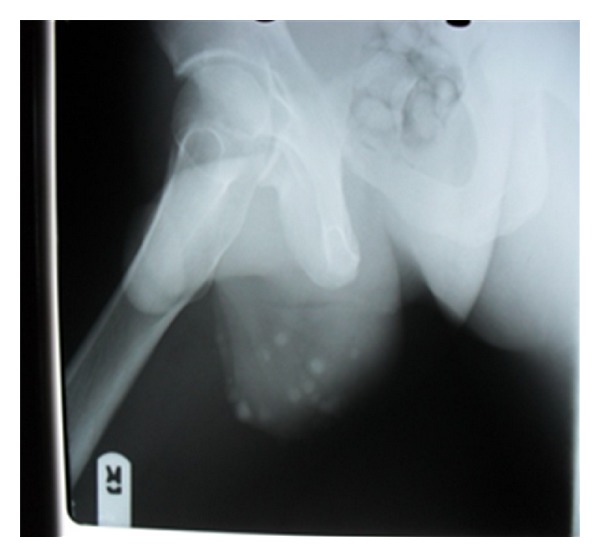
X-ray showing calcific scrotal nodules.
